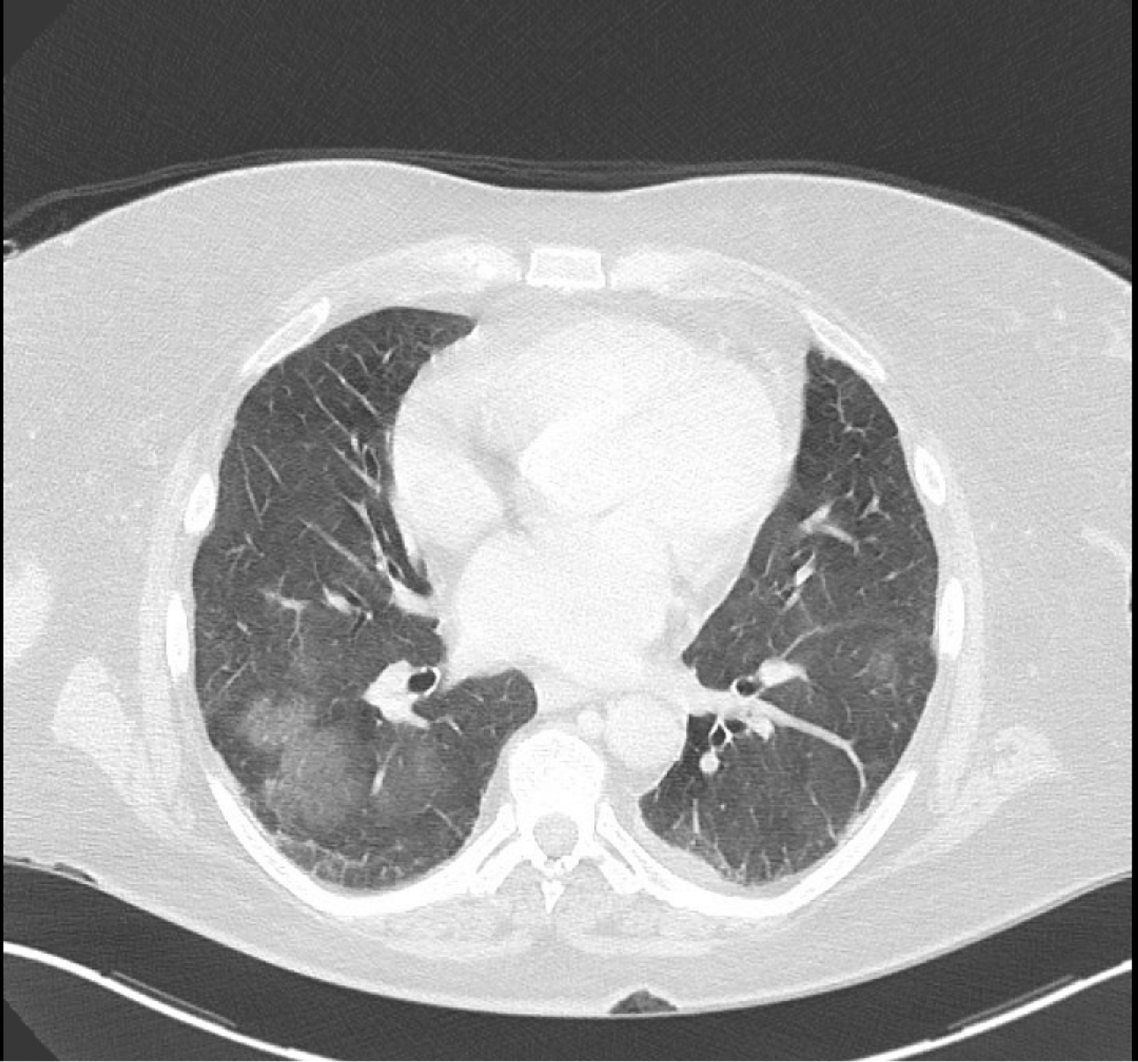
A computed tomography scan of the patient’s chest depicting bilateral central ground-glass opacities
